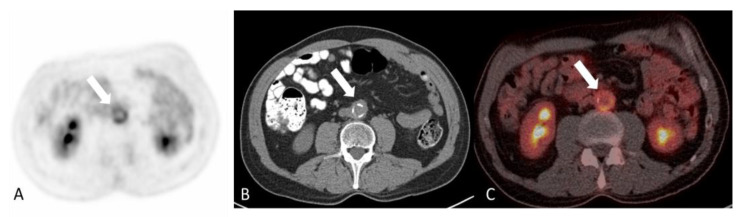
65-year-old woman with IgG4-related aortitis. Axial images of F-18 FDG attenuation corrected PET (A), CT without contrast (B) and fused PET/CT (C) demonstrating tracer avid concentric mural thickening involving the abdominal aorta (arrows) and underlying calcified atherosclerosis. No evidence of retroperitoneal fibrosis.
